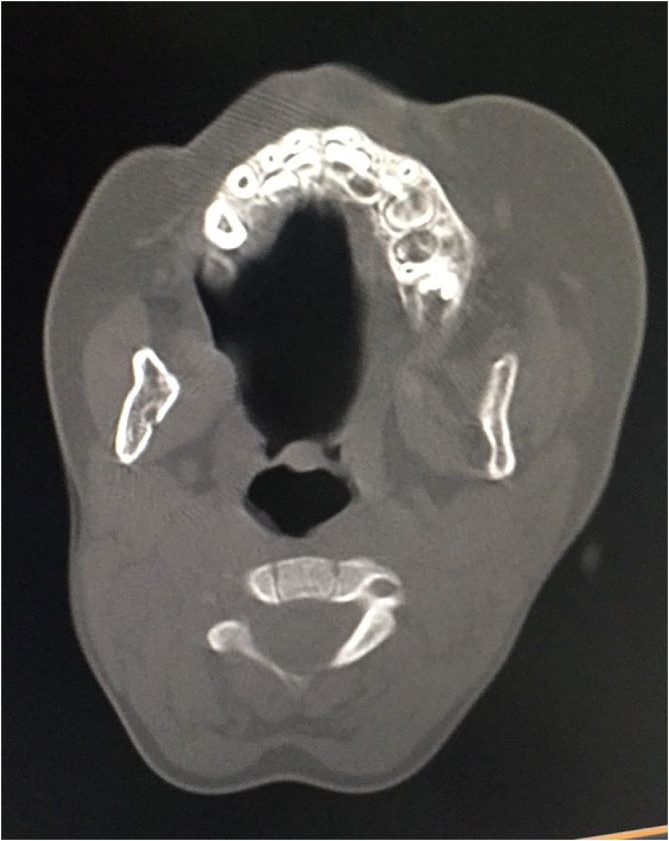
(CT) scan demonstrated a 4.5 cm nodular enhancing lesion with perilesional soft-tissue infiltration.
